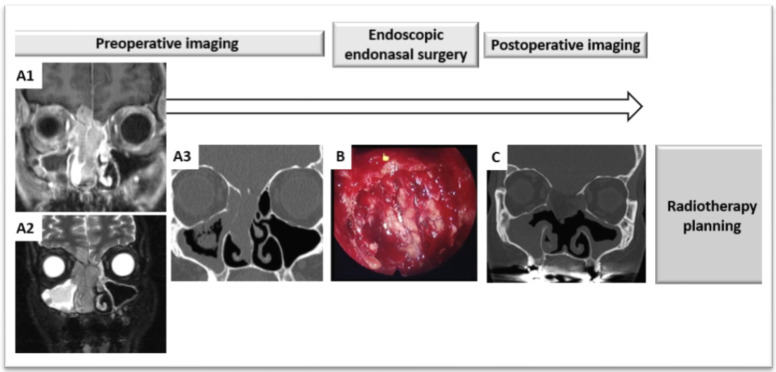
Perspectives of radiotherapy after endoscopic endonasal resection; a mini morbid multimodal strategy. Assessment of disease and therapeutic steps before radiotherapy planning in an olfactive neuroblastoma of the ethmoid. (A1): preoperative T1-gadolinium enhanced MR of the tumor, frontal view. (A2): preoperative T2 MR of the tumor, frontal view. (A3): preoperative CT of the tumor, frontal view. (B): endoscopic view of the tumor following resection. (C): postoperative CT at day 20 following tumor resection and base of skull reconstruction with abdominal fat flap, showing proper closure and no residual tumor.
